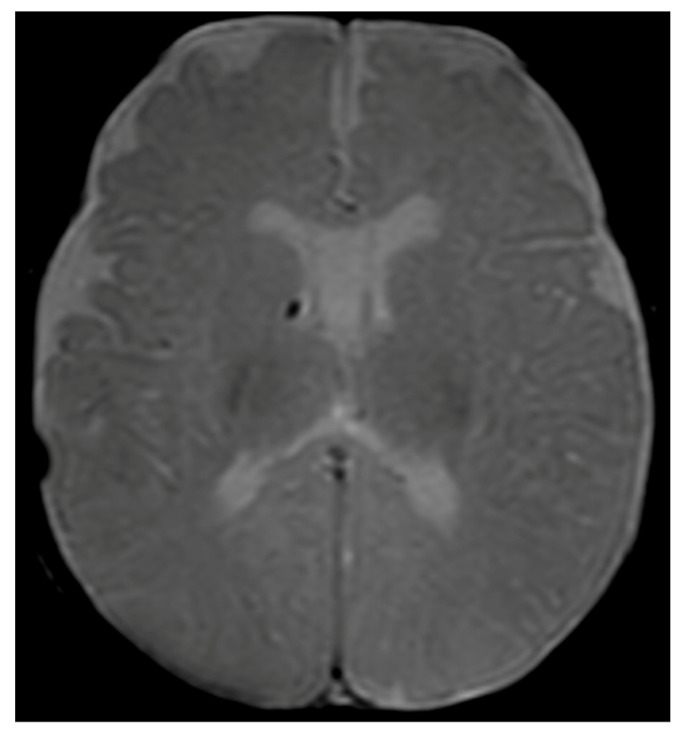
Brain MRI with axial T2-gradient echo image at 2 months and 3 weeks of life. Punctate susceptibility focus, suspicious for calcification/hemosiderin, in the right germinal matrix is visible.
